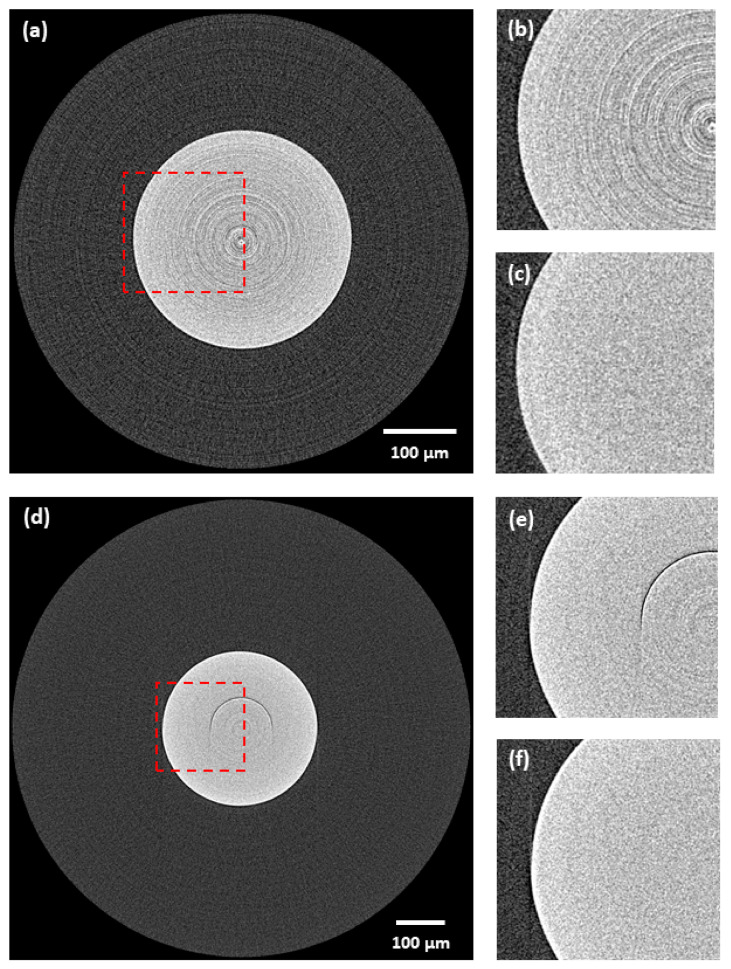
Demonstration of robustness of the proposed method to the used detection system—real CT data of ruby ball: (a) original tomogram (scientific complementary metal-oxide-semiconductor (sCMOS), without any correction)—red labelled area marks the central area visualized in following images; (b) detail (sCMOS)—original; (c) detail (sCMOS)—corrected by proposed method; (d) original tomogram (CCD, without any correction)—red labelled area marks the central area visualized in following images; (e) detail (CCD)—original; (f) detail (CCD)—corrected by proposed method. For visualization, the same contrast setting was used for all the images.
